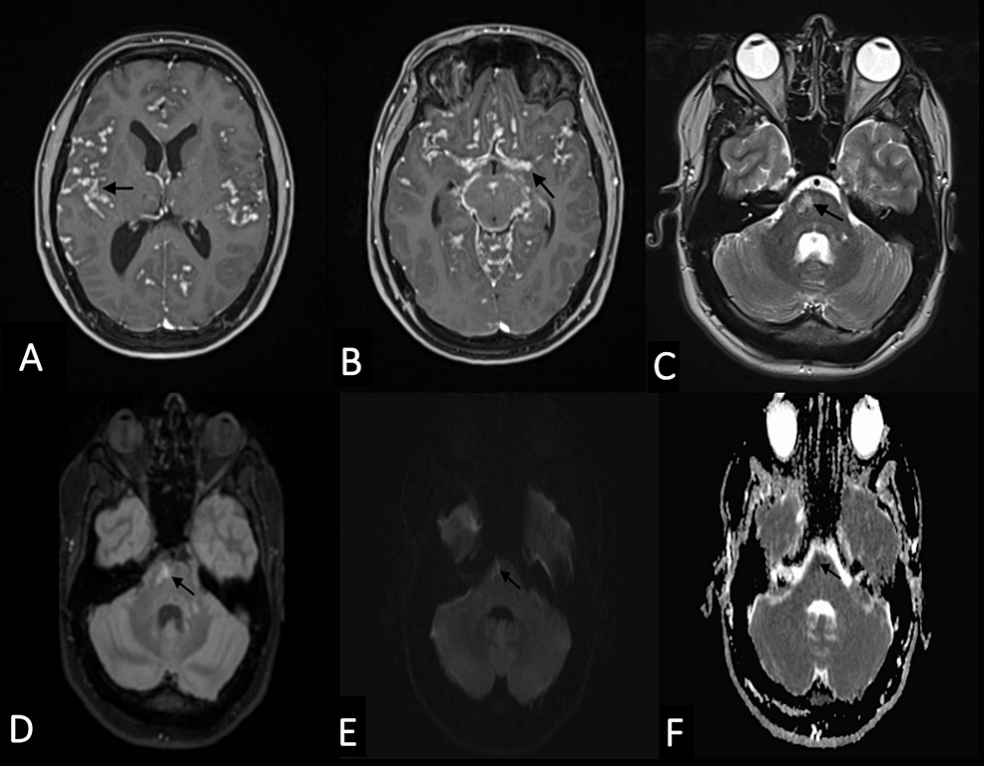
Brain MRI MRI showing the leptomeningeal nodular enhancement (black arrows) displayed on post-contrast axial T1WI (A,B). Recent pontic and brain stem ischemic lesions are demonstrated (black arrows), characterized by a hypersignal on sequences axial T2 (C) and axial FLAIR (D) with diffusion restriction (E,F). MRI: magnetic resonance imaging, T1WI: T1-weighted image, FLAIR: fluid-attenuated inversion recovery
